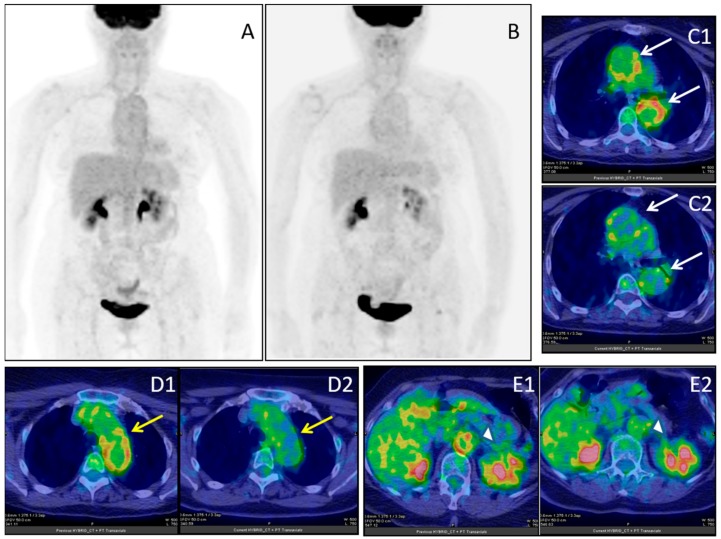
Female, 64 years-old; (^18^F)-FDG-PET/CT MIP in giant cell arteritis (GCA) before (A) and after (B) immuno-suppressive treatment; ascending and descending aorta (white arrows), before (C1) and after (C2) treatment; aortic arc (yellow arrow), before (D1) and after (D2) treatment; abdominal aorta (white arrow-head), before (E1) and after (E2) treatment.
